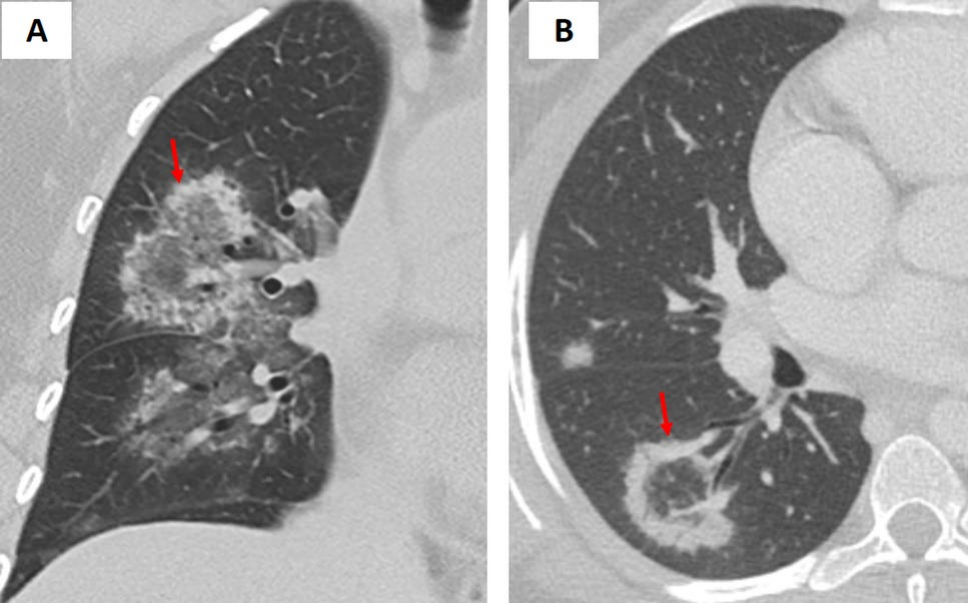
Atoll sign in a patient with malignant melanoma and immunotherapy-induced pneumonitis. A Coronal and (B) axial chest CT images demonstrate multiple peribronchial pulmonary opacities with the atoll sign (arrows). The causative agent was discontinued, and the opacities subsequently resolved
